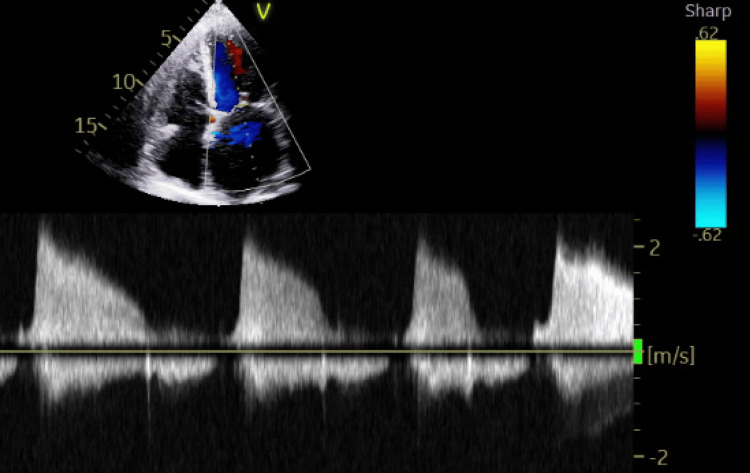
Transthoracic echocardiography in the apical four-chamber view demonstrating a mean transmitral pressure gradient of 9 mmHg
